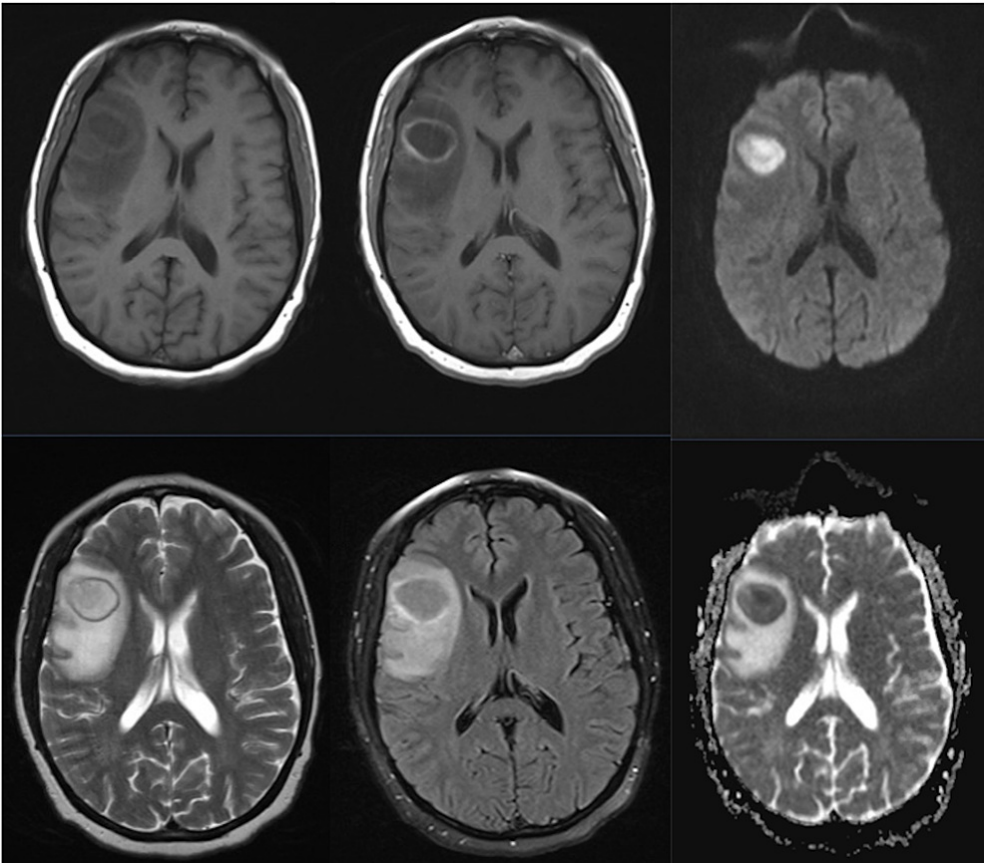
Preoperative MRI scan. Preoperative right frontal multiloculated, ring-enhancing lesion, with surrounding edema and restricted diffusion. First row: Preoperative T1- weighted, T1 + contrast, diffusion imaging. Second row: Preoperative T2-weighted, FLAIR, and ADC. ADC: apparent diffusion coefficient; FLAIR: fluid-attenuated inversion recovery; MRI: magnetic resonance imaging
